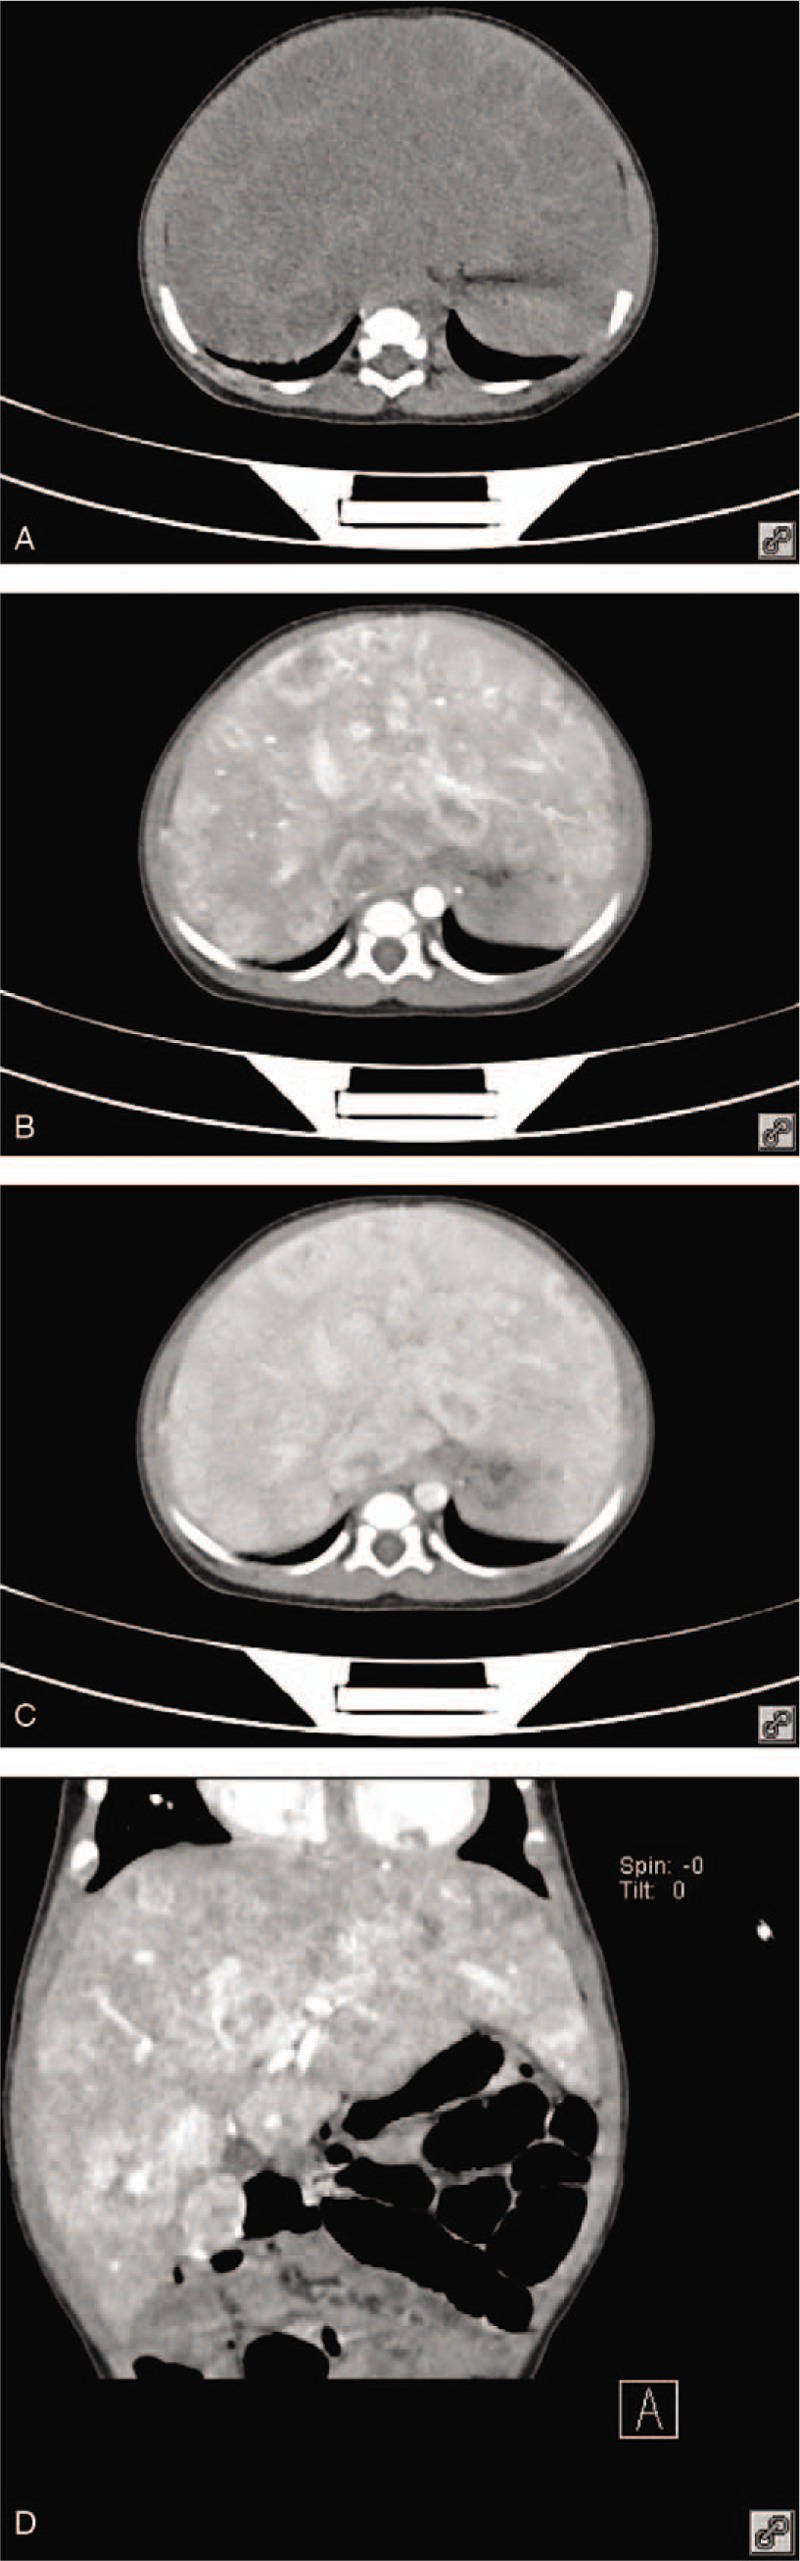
Computed tomography scan of the abdomen showed an enlarged, irregularly shaped liver with multiple low-density intrahepatic masses which present as enhance centripetally.
